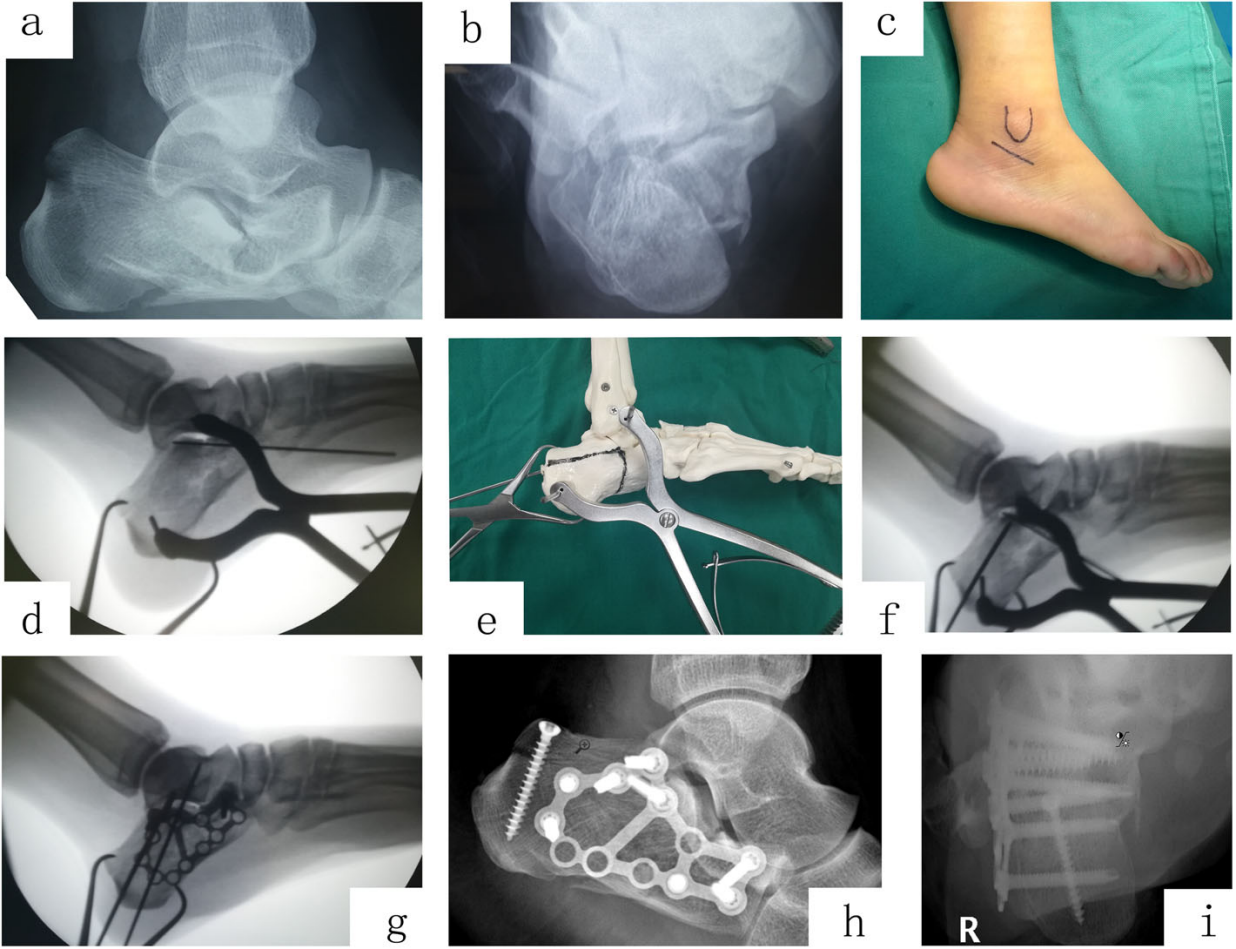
A 30-year-old male patient falls from a height of 1.5 m. Preoperative lateral (a) and axial (b) radiograph showed right calcaneus fracture with reduced Böhler angle (− 21°), length (75 mm), and height (61.1 mm), and width (59.3 mm). Steinmann pin retractor-assisted reduction with circle plate fixation via the sinus tarsi approach was used (c, d, e, f, g). Postoperative lateral (h) and axial (i) radiographs show obvious correction of the Böhler angle (32°), and improvements in calcaneal length (84.8 mm), height (80.5 mm), and width (40.4 mm). AOFAS score was 75 at the last follow-up
